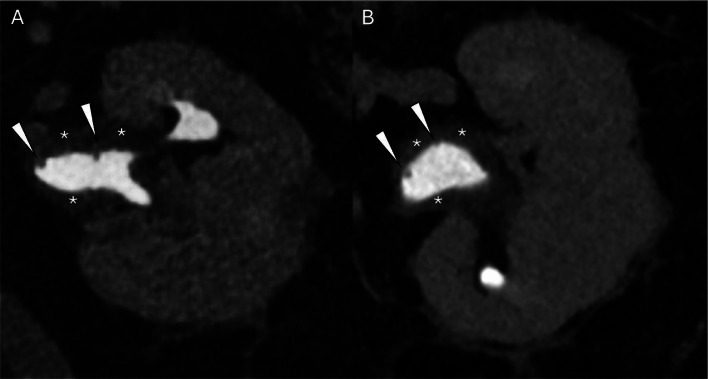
Radiology findings: Axial (A) and coronal (B) slices of CT urography in excretory phase at the level of the left kidney demonstrate extensive urothelial thickening (*) of the left renal pelvis and proximal ureter (not shown) with subtle nodular filling defects (arrowheads).
